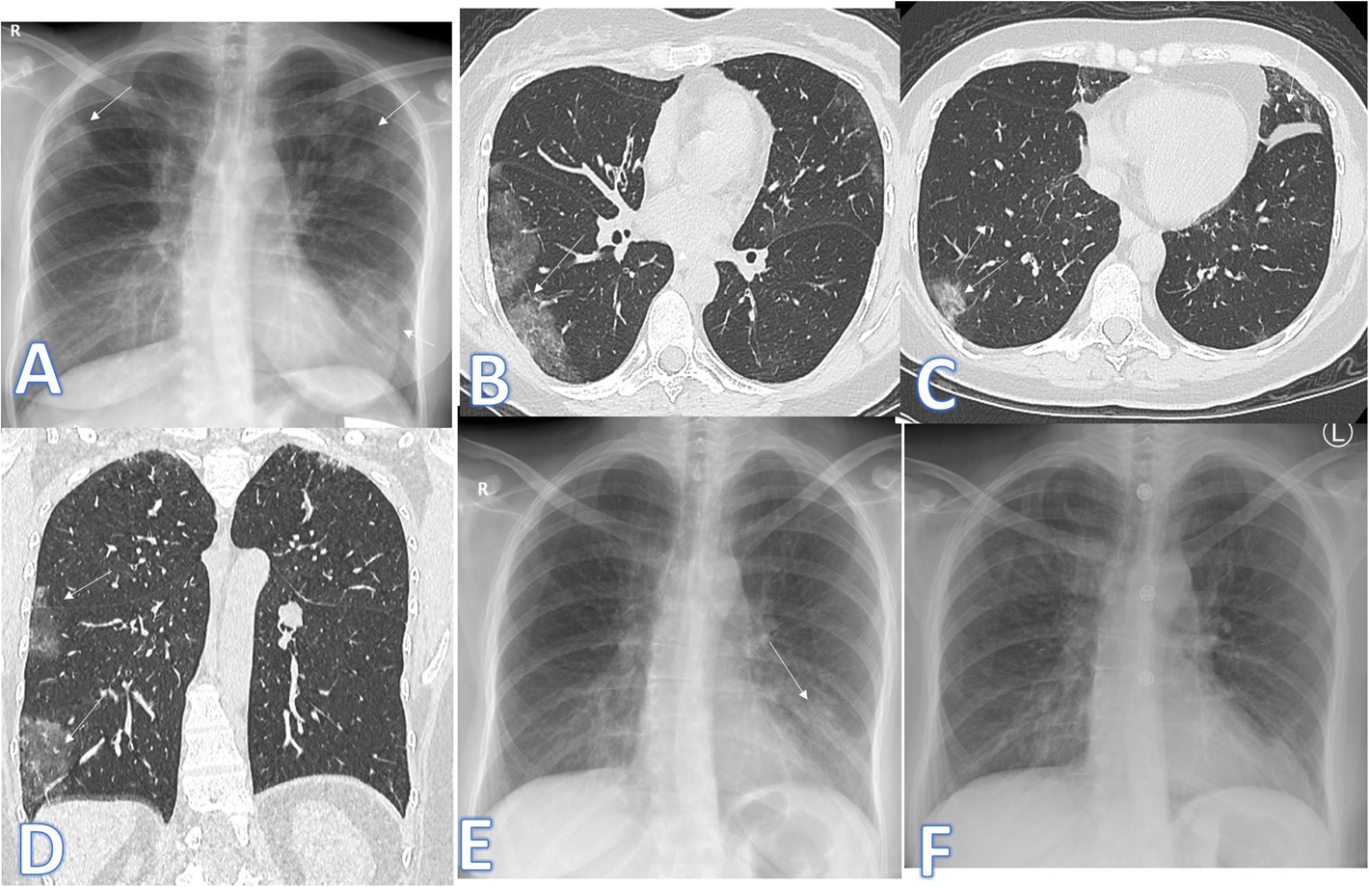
A 38-year-old female patient with fever and cough. Initial X-ray (a) showed bilateral small air space opacities (arrows). On CT chest, there was bilateral peripheral GGO (arrows). X-ray done 1 year before (e), and there was a left lower lobe air space opacity which was resolved on X-ray (f) 6 months later (fleeting). The diagnosis was Loffler’s pneumonia
